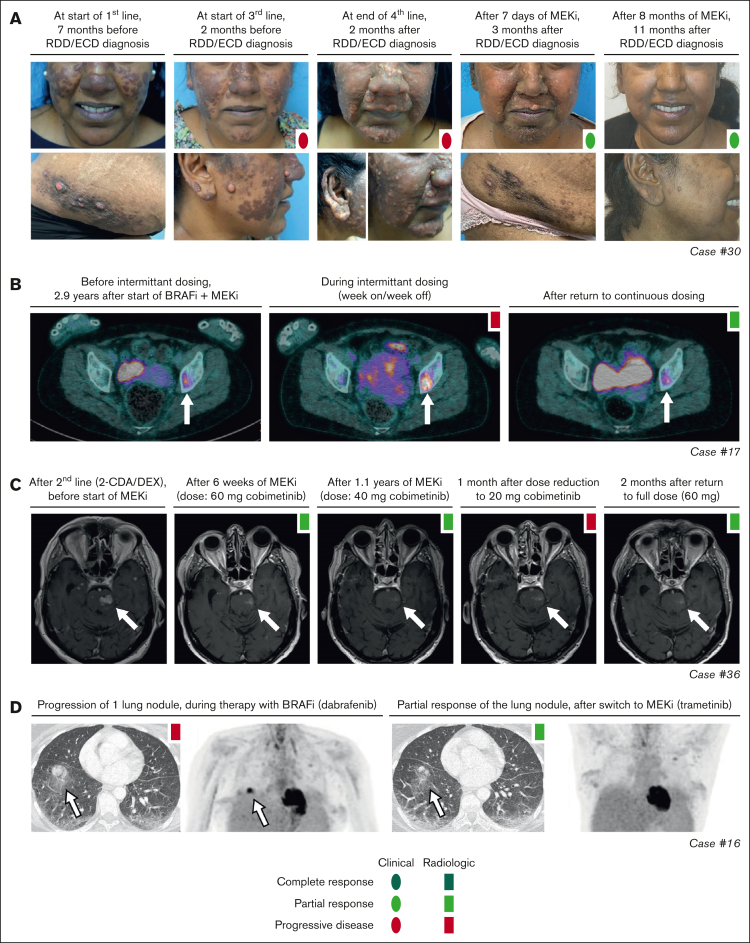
Response and rare disease progression on targeted therapy. (A) Photographs showing response of skin lesions in case 30 with RDD/ECD to conventional therapies and targeted treatment with cobimetinib. (B) Axial PET-computed tomography (CT) images of case 17 with ECD showing increased fluorodeoxyglucose (FDG) uptake of a left acetabular lesion (indicated by a white arrow) during intermittent dosing of dabrafenib/trametinib. After returning to continuous therapy, PET-CT showed decreased FDG uptake in the acetabular lesion, consistent with a recaptured PR. (C) Axial T1-weighted gadolinium-enhanced MRI images of case 36 with CNS-RDD showing an initial PR of contrast-enhanced brain lesions to treatment with cobimetinib, and subsequent progression of brain stem lesions after dose reduction to 20 mg/d. After return to full dose (60 mg/d), lesions decreased again, indicating a recaptured response. (D) Axial CT and PET images of case 16 with ECD showing progression of an FDG-avid lung nodule during treatment with dabrafenib, and partial resolution of this nodule after switch to treatment with trametinib. 2-CDA, cladribine; DEX, dexamethasone; BRAFi, BRAF inhibitor; MEKi, MEK inhibitor.
